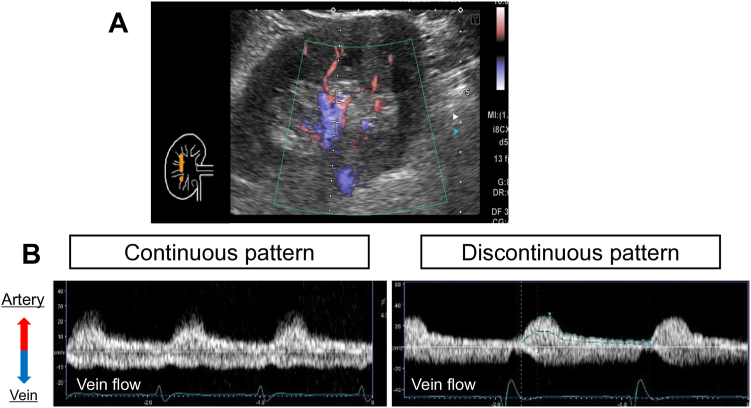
Intrarenal venous flow (IRVF) assessment with intrarenal Doppler ultrasonography. (A) Color Doppler imaging of IRVF. (B) Representative Doppler waveforms of IRVF, showing (left) a continuous pattern and (right) a discontinuous pattern.
